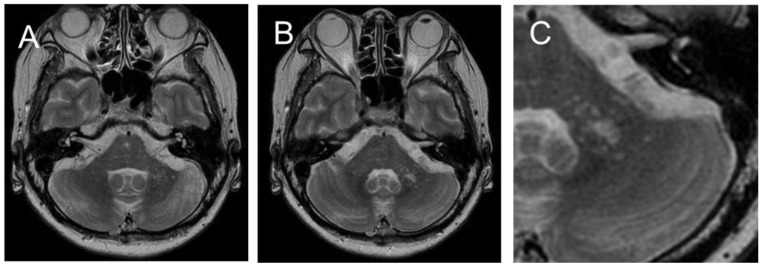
MRI on day 39 revealed increasing spotty lesions in the left peduncle that became centrally clustered, with a spreading spotty lesion surrounding the central area (A,B). ((C): Enlarged image of (B)). No cerebellar cortex atrophy was observed.
